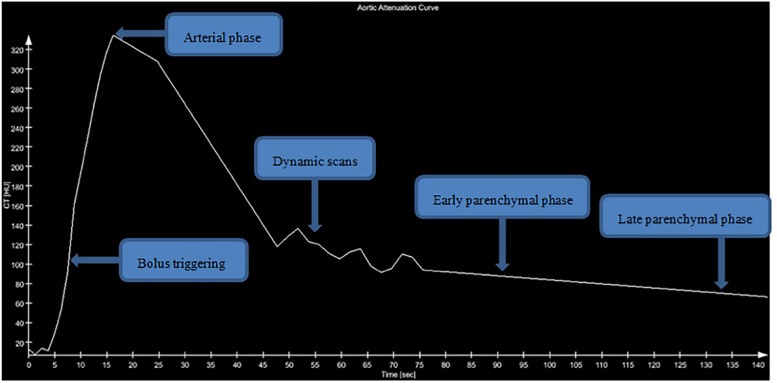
Aortic attenuation curve. Graph shows aortic attenuation curve of a 35-year-old man with unilateral renal tumor. Five clusters of data points were measured with CT. The area under the curve was determined graphically.
